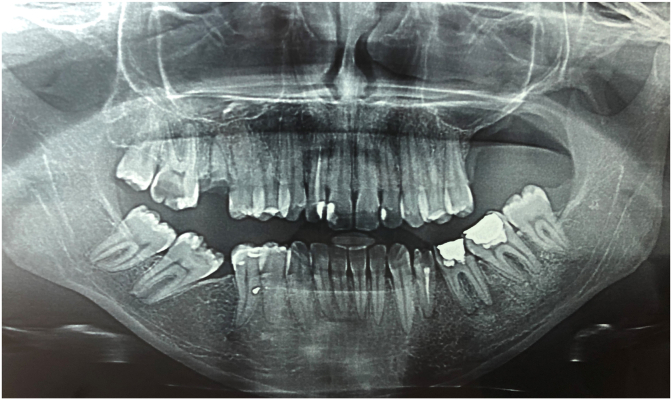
Orthopantomogram view showing maxillectomy at the left maxillary bone after surgery.
